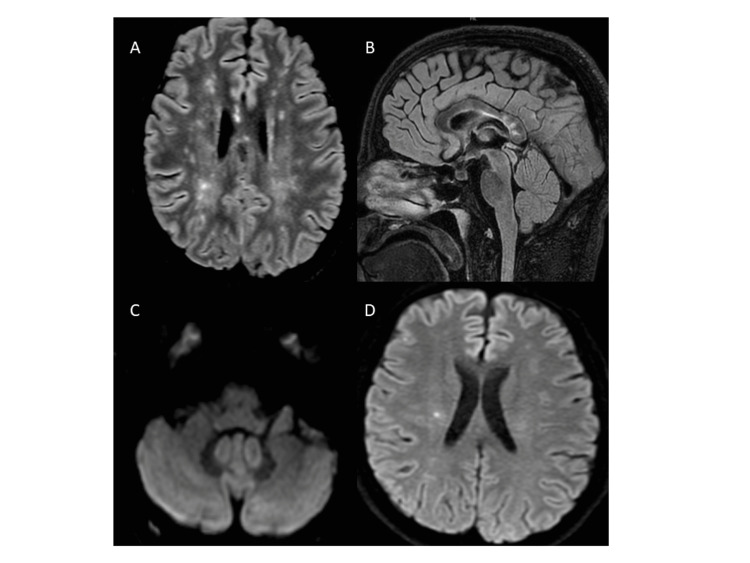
Cerebral MRI images three months after discharge (A-D) (A, B) Reduction in the number and size of the lesions on axial and sagittal FLAIR sections. (C, D) Only a few punctate lesions can be observed on DWI sequence.
